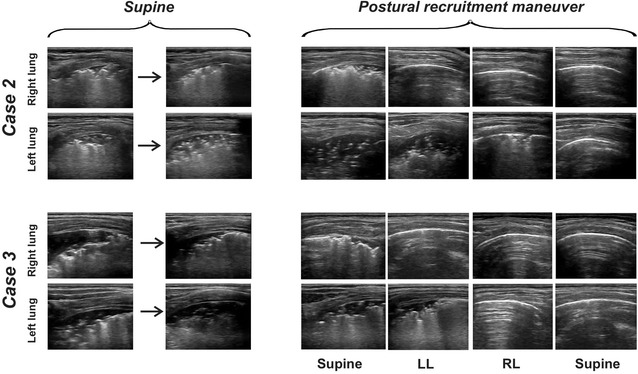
Lung ultrasound images of postural recruitment in case 2 and 3
